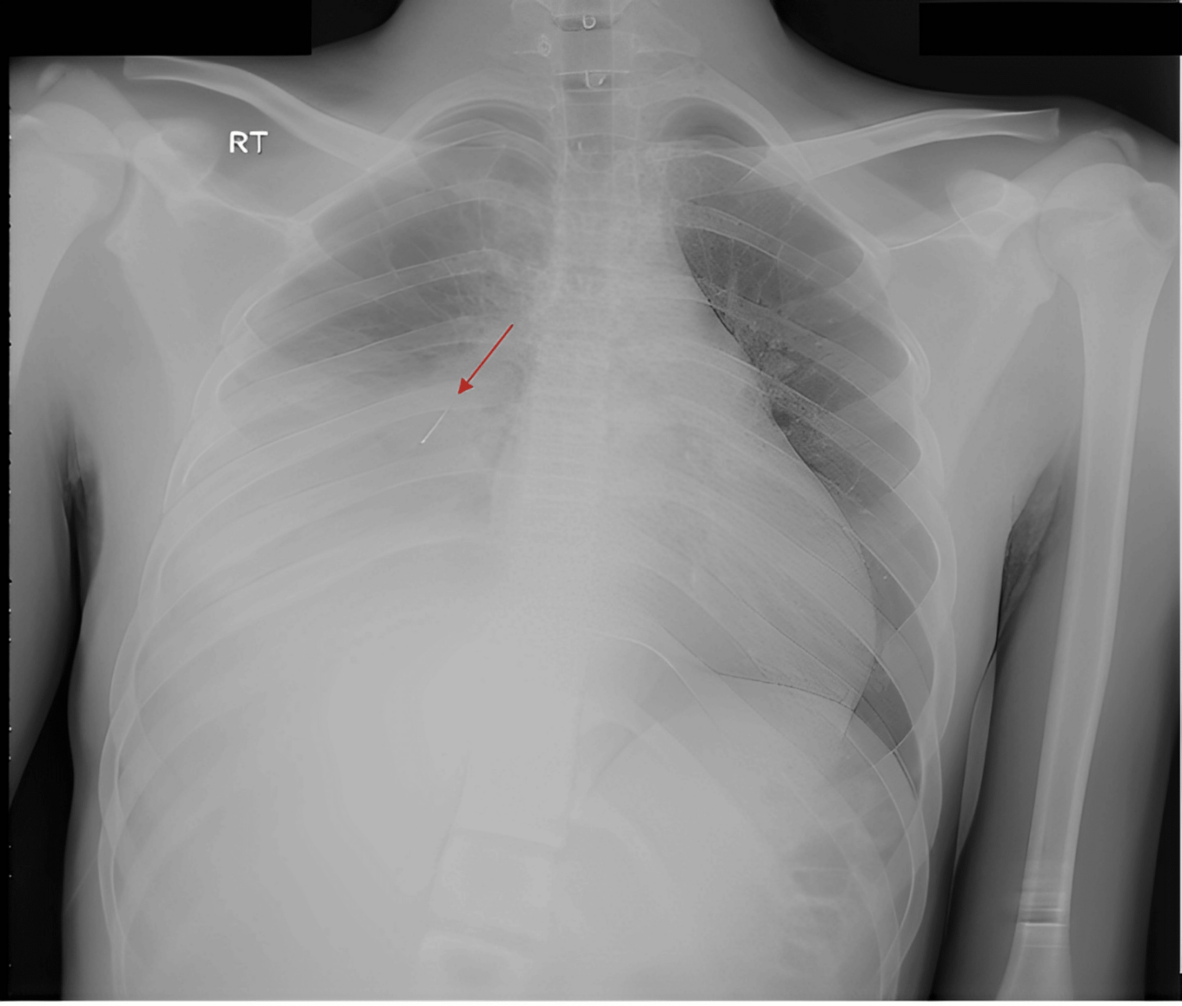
Chest radiograph showing a sharp radiopaque foreign body in the right bronchus intermedius
